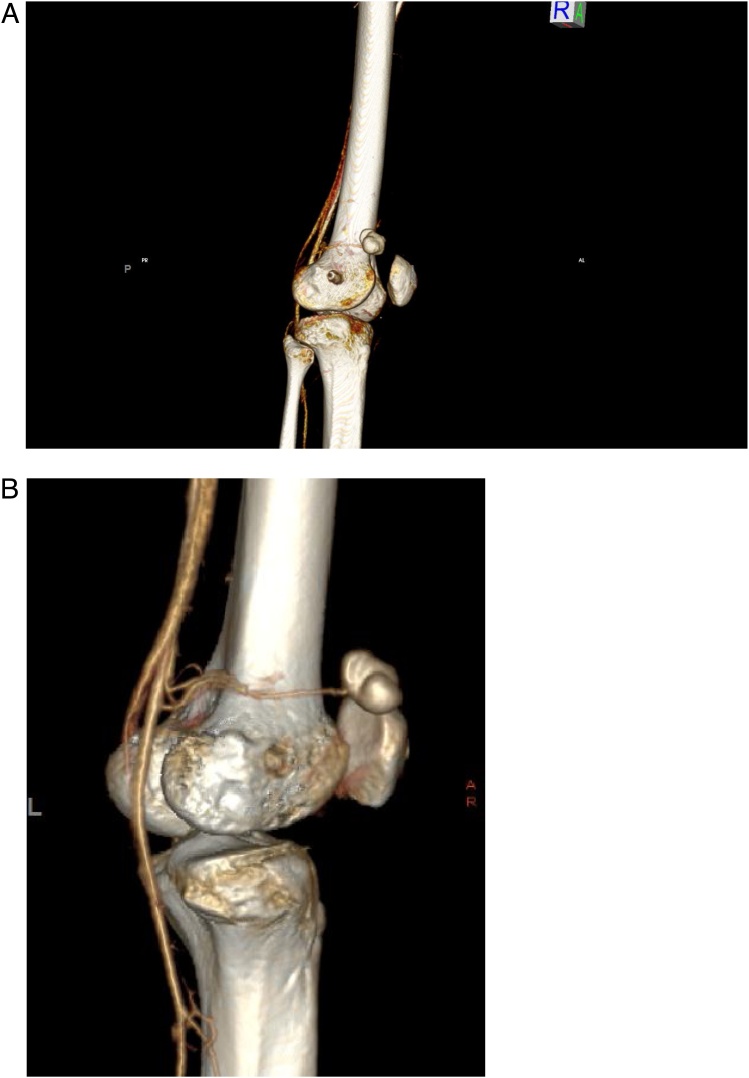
A Lateral view of a 3D Reconstructed CT with the visualization of the pseudoaneurysm depending of the supero-lateral genicular artery. B ¾ view with the popliteal artery and the pseudoaneurysm of its branch.
